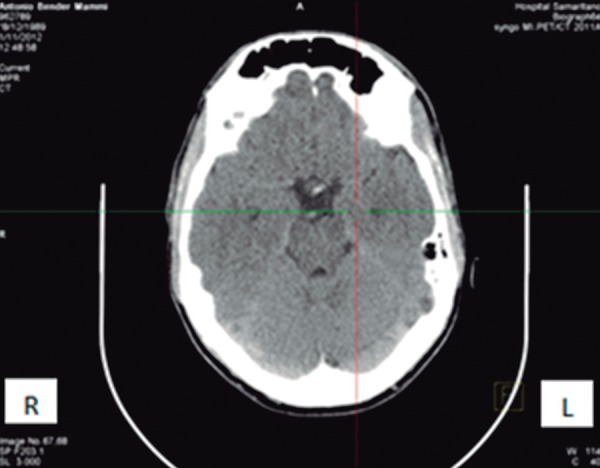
Cranial CT scan. No abnormalities were found.
